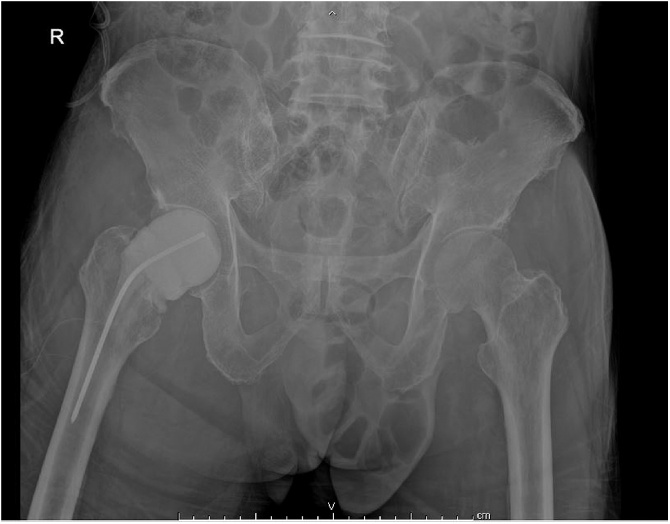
Plain radiograph after the first operation.
